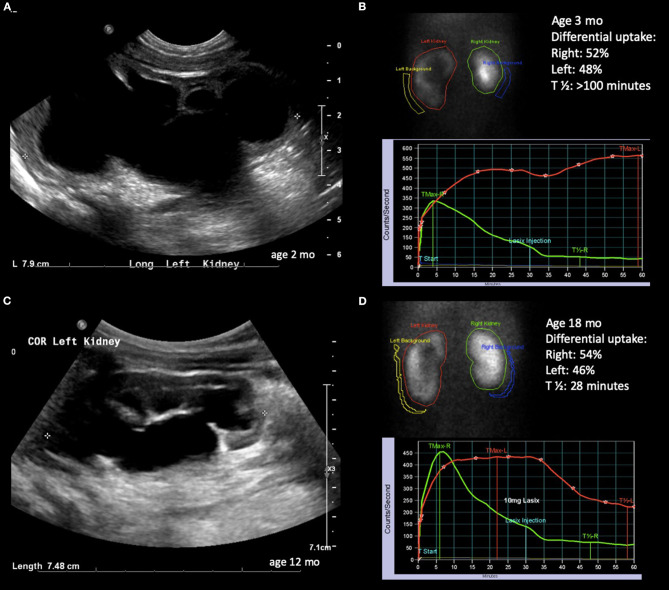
Radiological history of an infant with severe hydronephrosis secondary to UPJ obstruction and biphasic diuretic curve. (A) Ultrasound confirming SFU grade 4 hydronephrosis at 2 months of age. (B) Diuretic renogram showing symmetrical uptake with biphasic washout curve. (C) Ultrasound at 12 months of age showing slightly improving but still SFU grade 4 hydronephrosis. (D) Improved washout curve on diuretic renogram, but still with delayed emptying.
